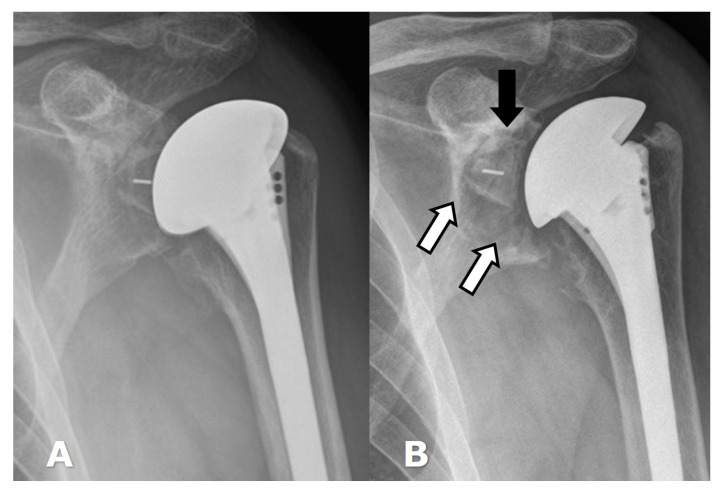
(A). Frontal radiograph in a patient with a normal-appearing ATSA. (B). Frontal radiograph several years later when patient presented with shoulder pain, showing lucency (white arrows) along the glenoid component, with slight superior rotation (black arrow) of the glenoid component within the bone, consistent with glenoid component loosening.
